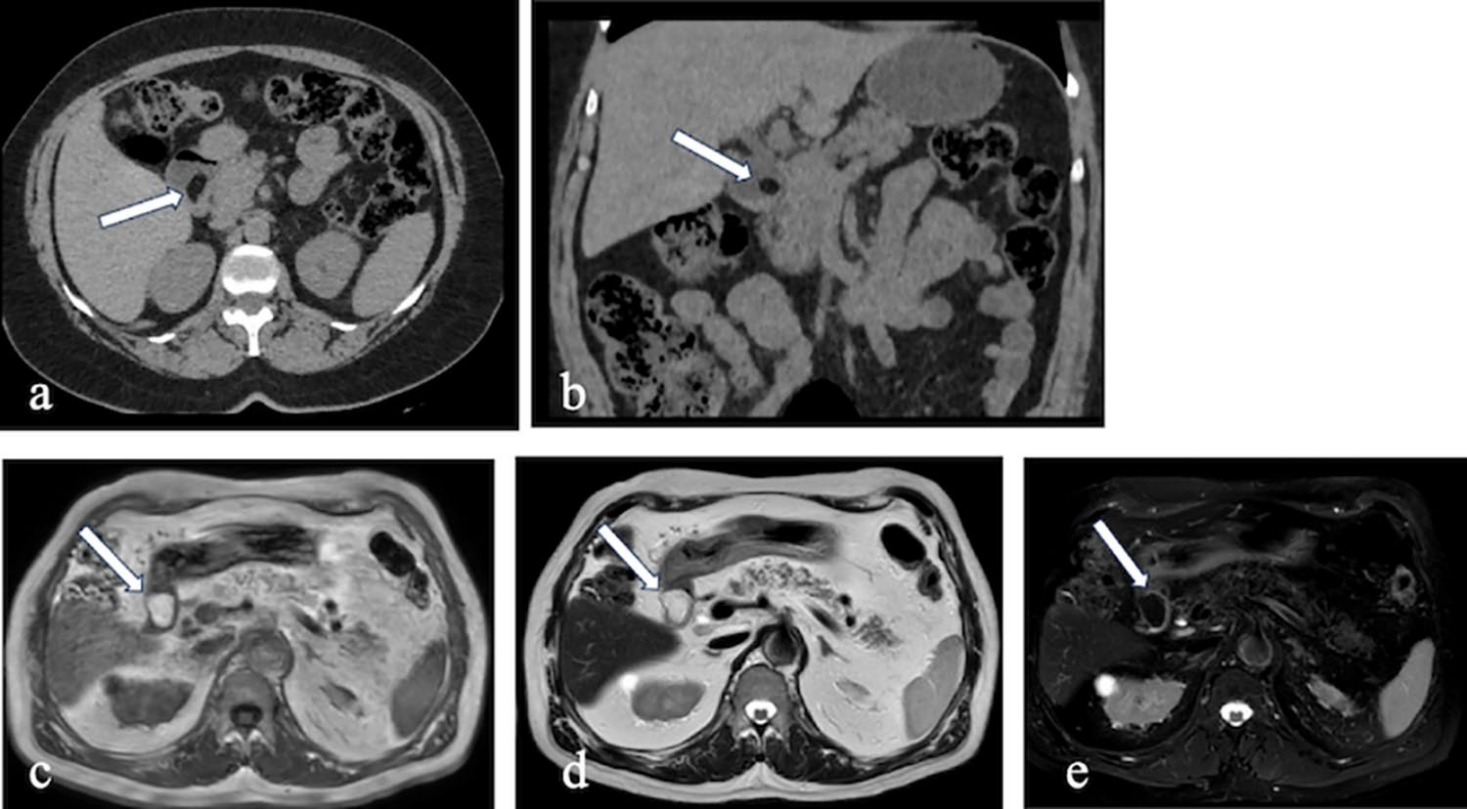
a. Axial and b. Coronal non-enhanced CT images of a 37-year-old male patient with a duodenal lipoma, exhibiting fat attenuation. MRI images c. T1-weighted, d. T2-weighted, and e. fat-saturated T2-weighted of another patient (58-year-old female) showing a lesion with high signal intensity on T1- and T2-weighted images, with signal suppression on fat-saturated sequences (arrows)
